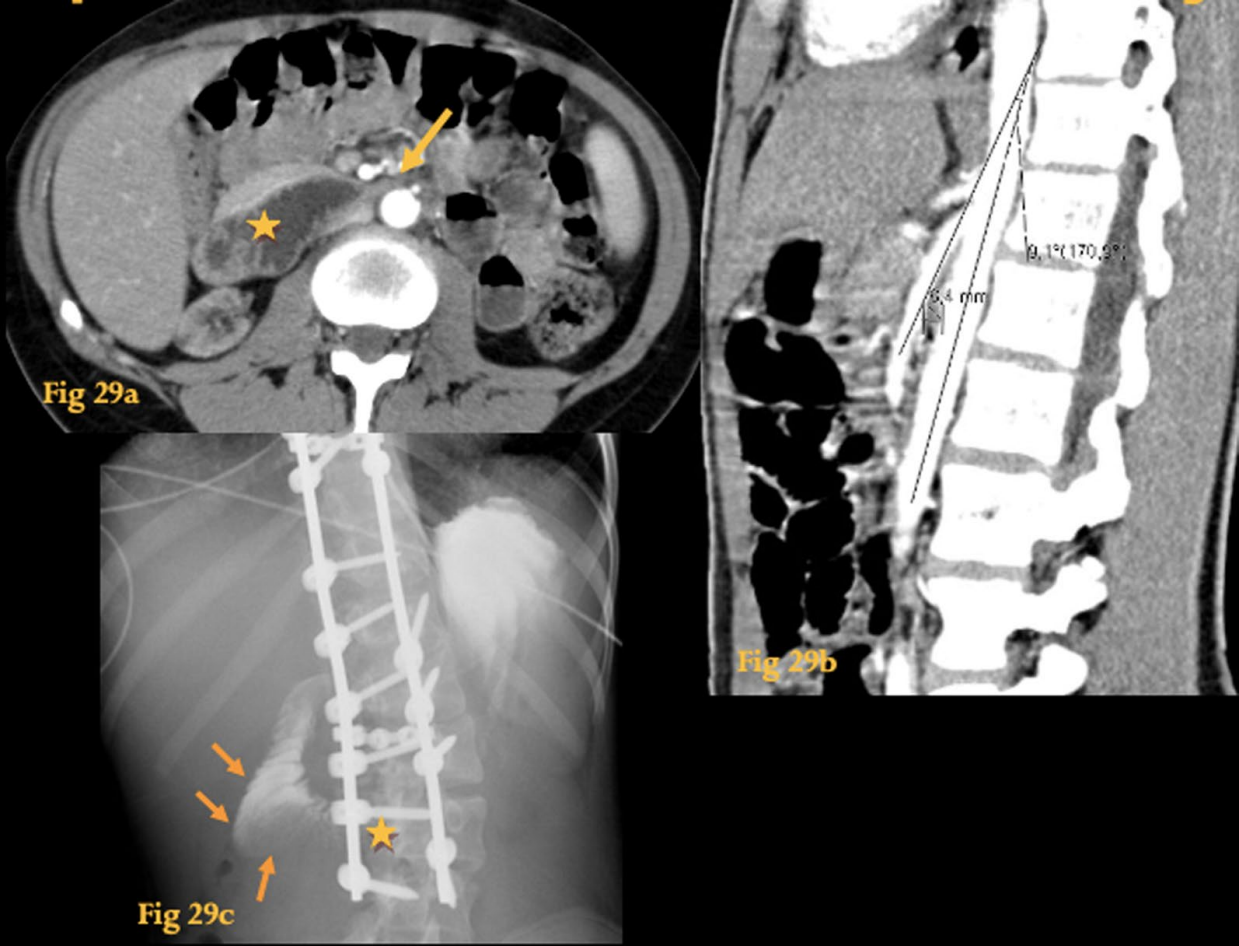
Superior mesenteric artery syndrome. Arterial phase of an abdominal CT study of a female adolescent (a) shows dilatation of the duodenum (asterisk) upstream to the point where it crosses the SMA and the aorta (arrow). Note paucity of abdominal fat. Oblique sagittal reformation of another patient (b) shows reduction of the normal aortomesenteric angle (9º) and aortomesenteric distance (6 mm). Fluoroscopic series of a 10-year-old girl after surgical correction of kyphosis c shows obstruction at D3 with delayed passage of contrast through the stenosis (asterisk) and proximal dilatation of D2(arrows). This was due to SMA syndrome caused by lengthening of the vertebral column
